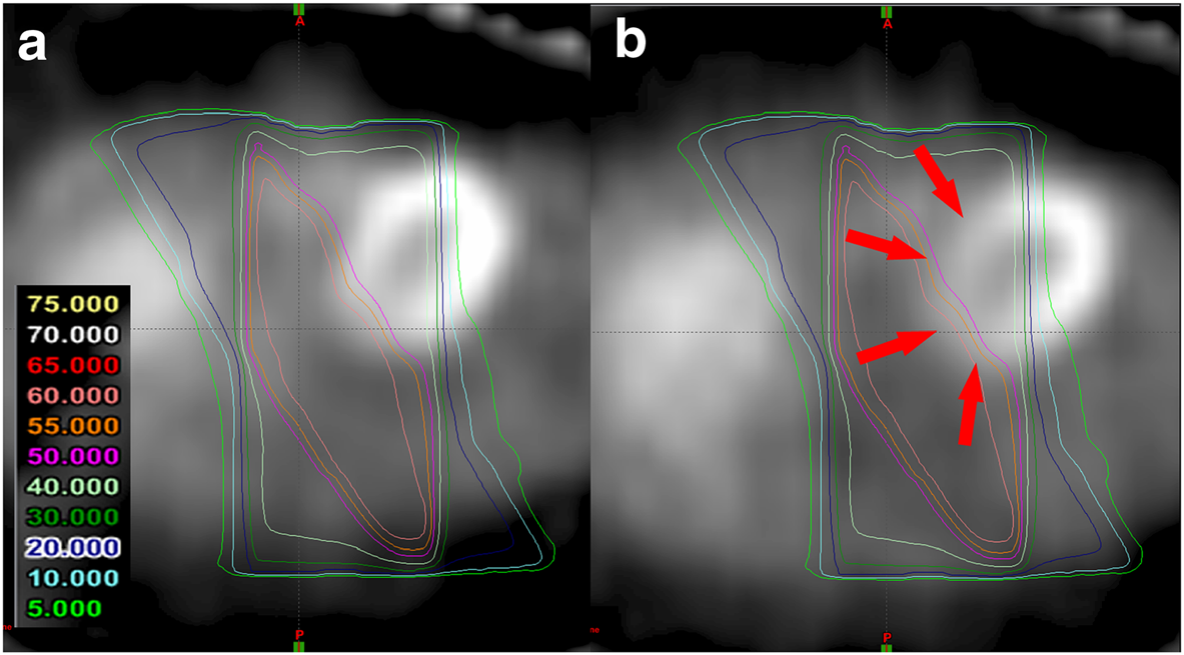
Findings of single photon emission computed tomography (SPECT) in Case 5. Reduced uptake corresponding to anterior-posterior fields was observed (red arrow). (a) pre-RT SPECT. (b) post-RT SPECT.
